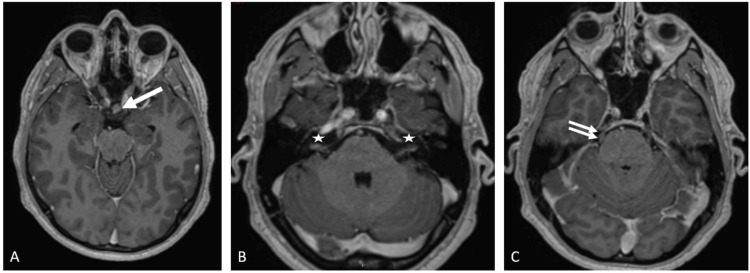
MRI brain axial T1 MPRAGE post-contrast images showing enhancing nodules in suprasellar cistern (A, arrow), bilateral VII-VIII nerve complex (B, stars), and trigeminal nerves (C, two small arrows along right trigeminal nerve).
